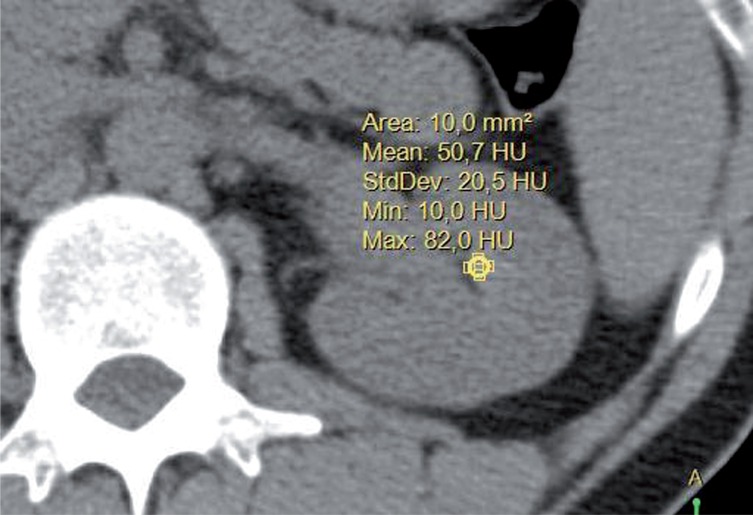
CT with measurement of renal papilla with an area of 10mm2, obtaining the mean of this area in Hounsfield units.
